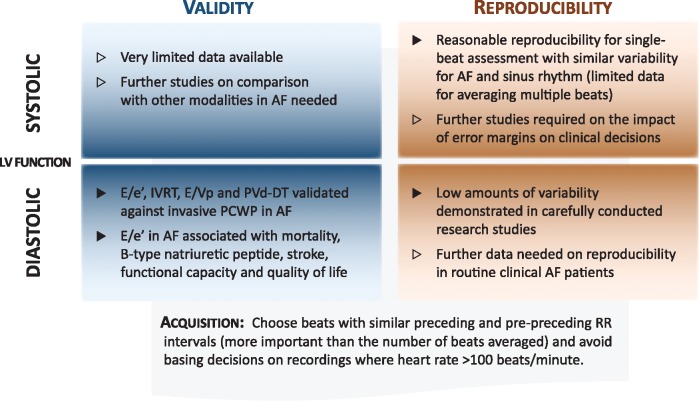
Summary of findings for echocardiography in AF. AF, atrial fibrillation; E/e’, ratio of mitral peak E velocity and tissue Doppler early diastolic filling e’; E/Vp, ratio of mitral peak E velocity and the velocity of diastolic flow propagation; IVRT, isovolumic relaxation time; PCWP, pulmonary capillary wedge pressure; PVd-DT, pulmonary venous diastolic flow deceleration time.
